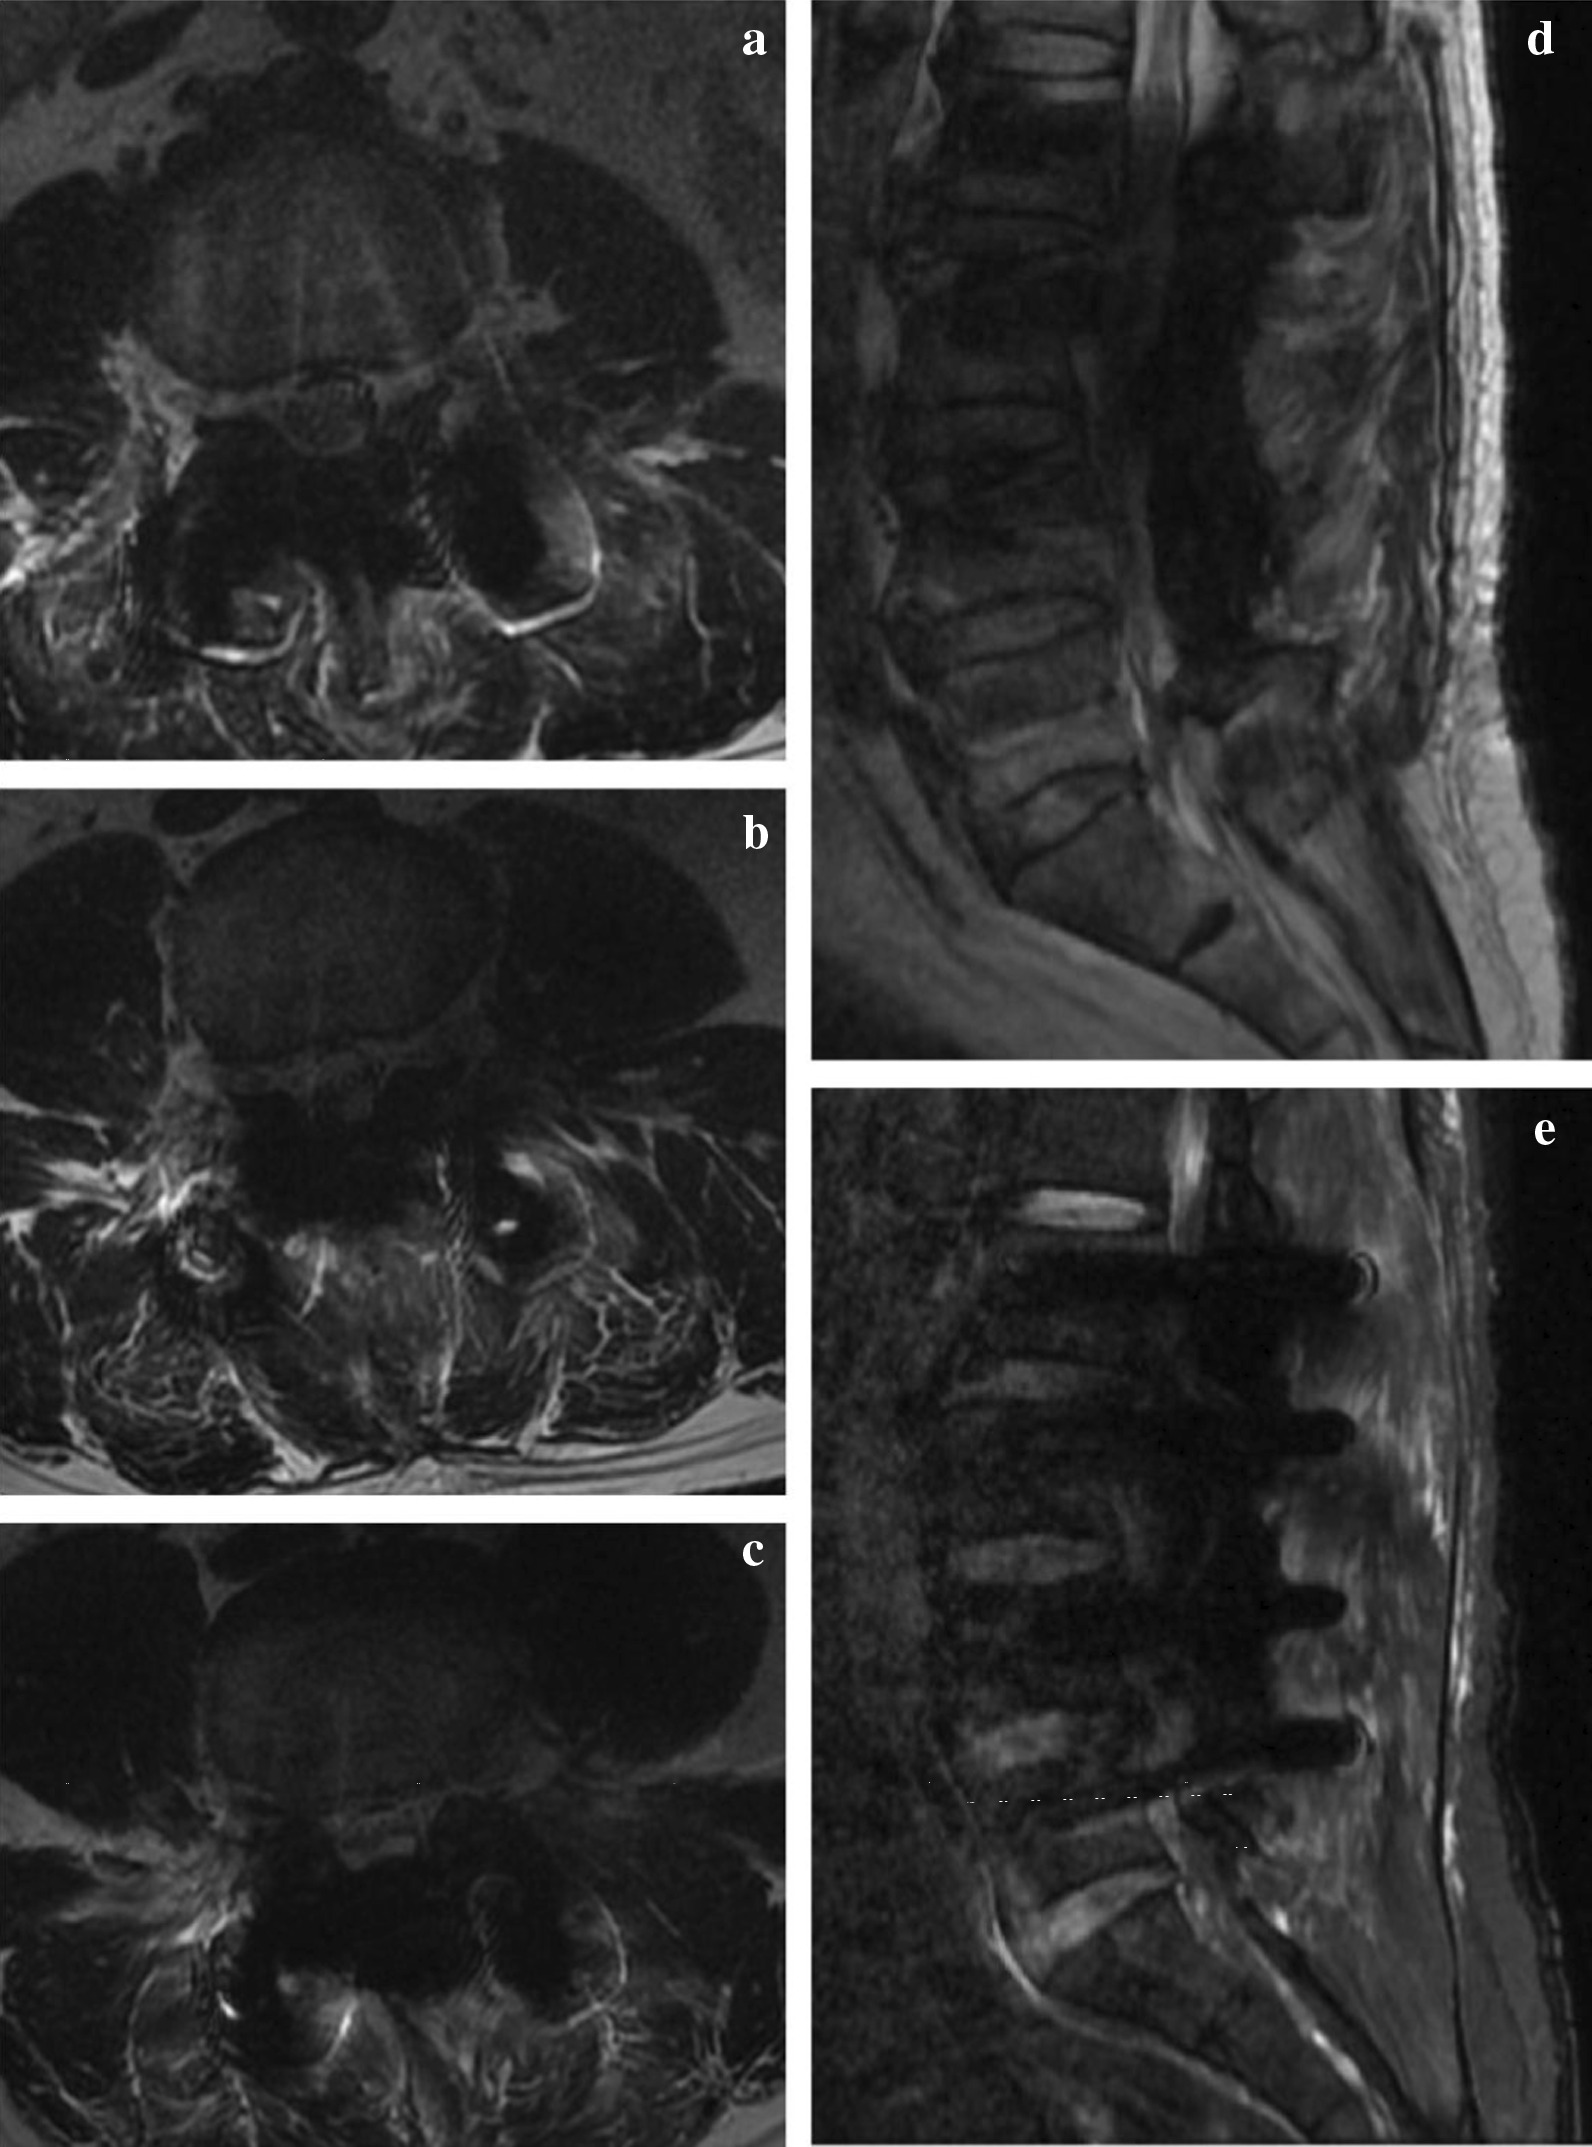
Immediate postoperative MRI. Immediate postoperative MRI image shows complete cancelation of extral adipose tissue and adequate decompression. a Axial image of L2/3. b Axial image of L3/4. c Axial image of L4/5. d T2-weighted sagittal image. e Fat suppression image
